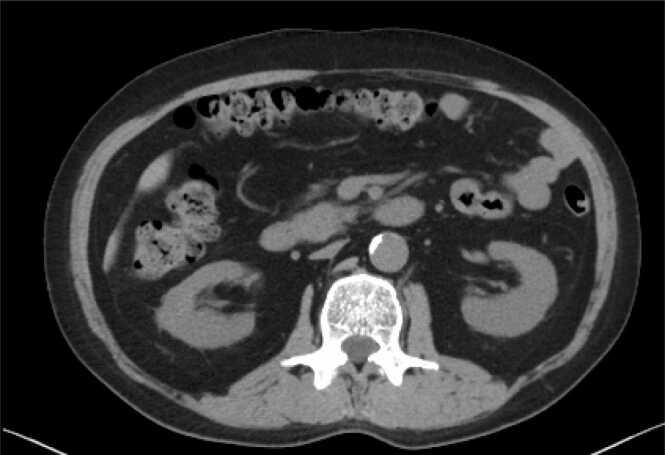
Preoperative Computed tomographic (CT) scan before the second lung transplantation. No obvious tumor lesions were detected in the pancreatic head.
